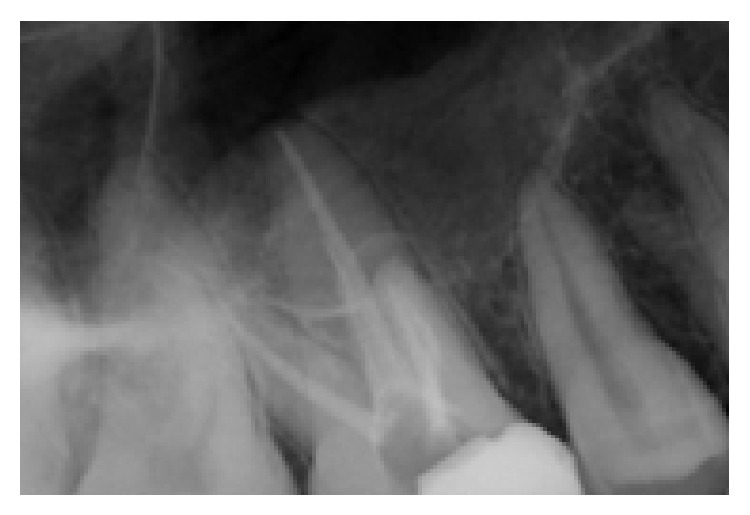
Postobturation radiograph showing all 3 MB canals exiting as single canal.
